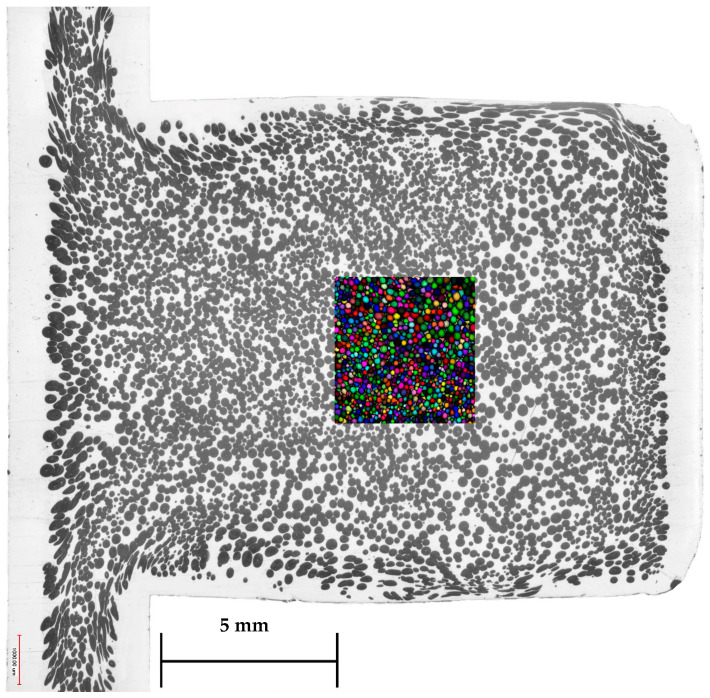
Measuring volume (colored area) of the X-ray microtomography images with the dimensions of 3000 µm × 3000 µm × 3800 µm.
